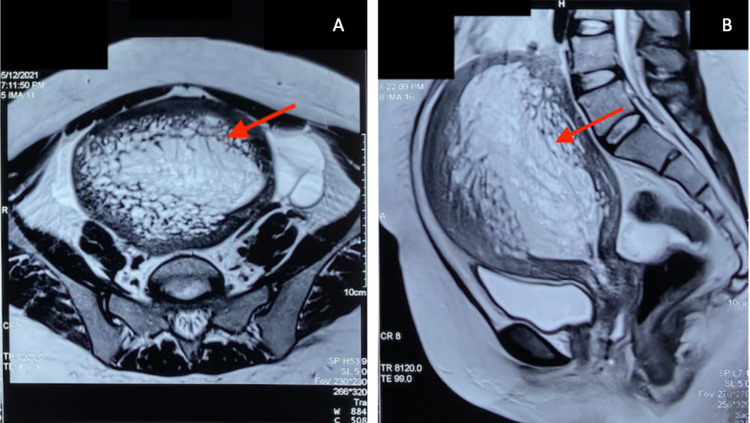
T2-weighted MRI scans revealing adenomyosis seen as enlarged junctional zone with indistinct margins infiltrating into the myometrium covered with cystic areas (arrowheads) (A) axial view (B) sagittal view. MRI: magnetic resonance imaging
